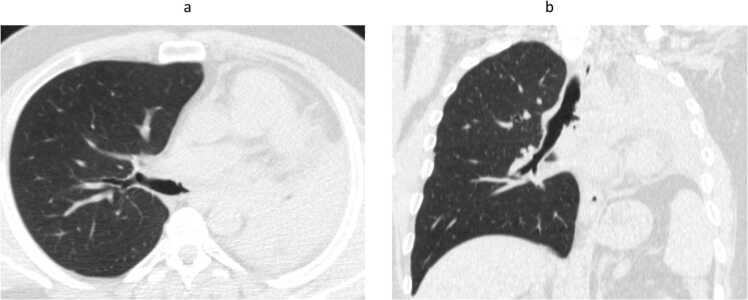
Axial and coronal CT images in lung window at the level of the carina. Axial (a)and coronal (b) CT images in lung window at the level of the carina showing total obliteration of the left main bronchus by an iso dense lesion, with subsequent total collapse of the left lung and minimal left pleural effusion.
